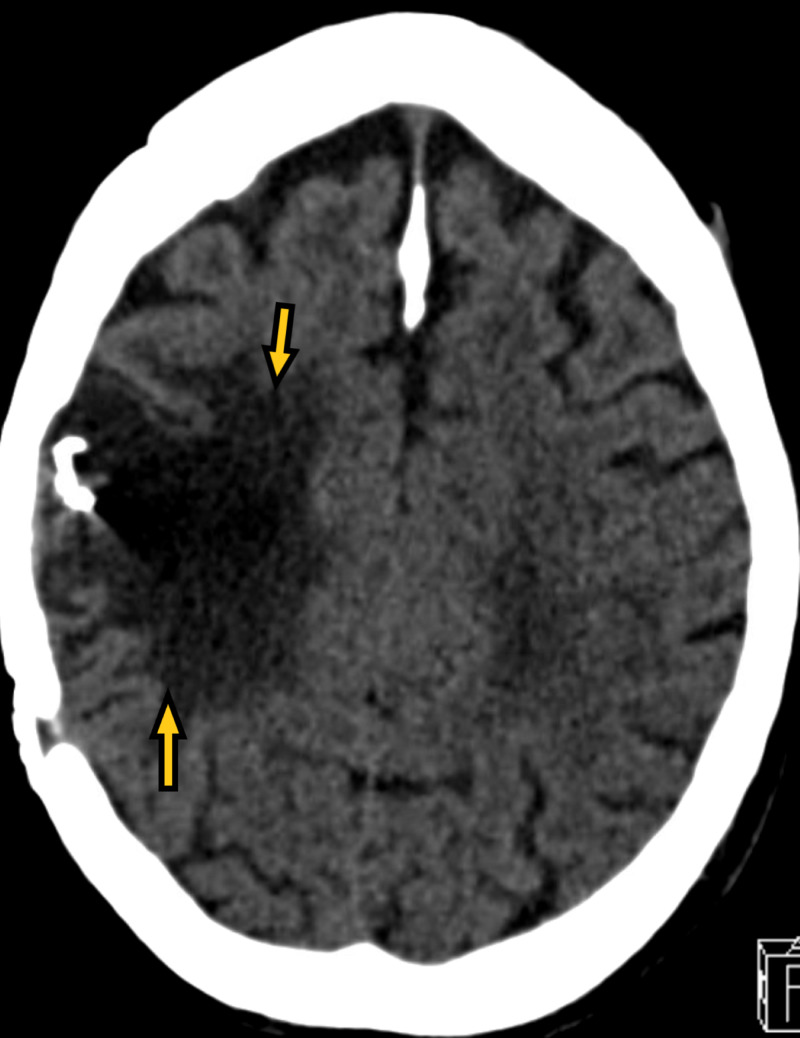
CT of the brain showing right sided encephalomalacia. CT of the brain denotes CT of the head.
